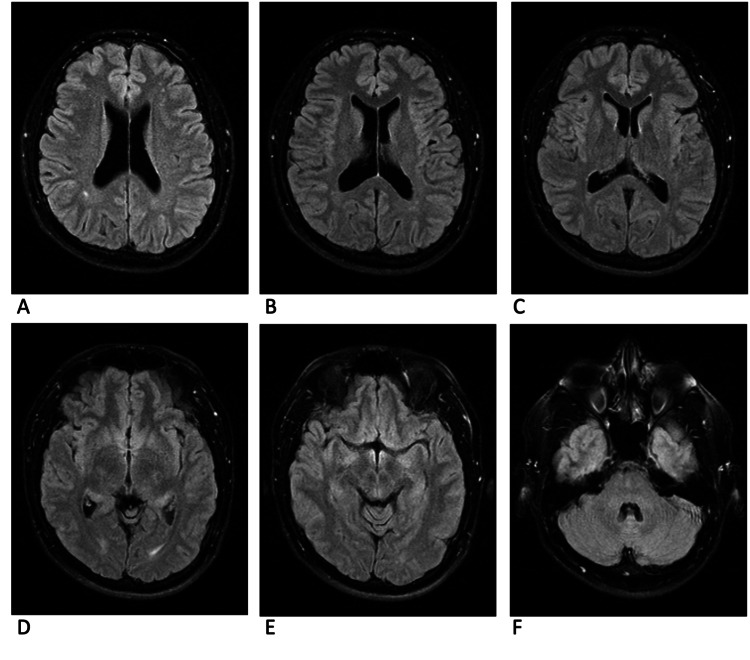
MRI brain T2 flair sequence 31 weeks after dual antiparasitic treatment Significant reduction of cyst inflammation is demonstrated (panes A-D). The lesion in the right posterior membrane is nearly resolved and has minimal inflammation (pane E).
